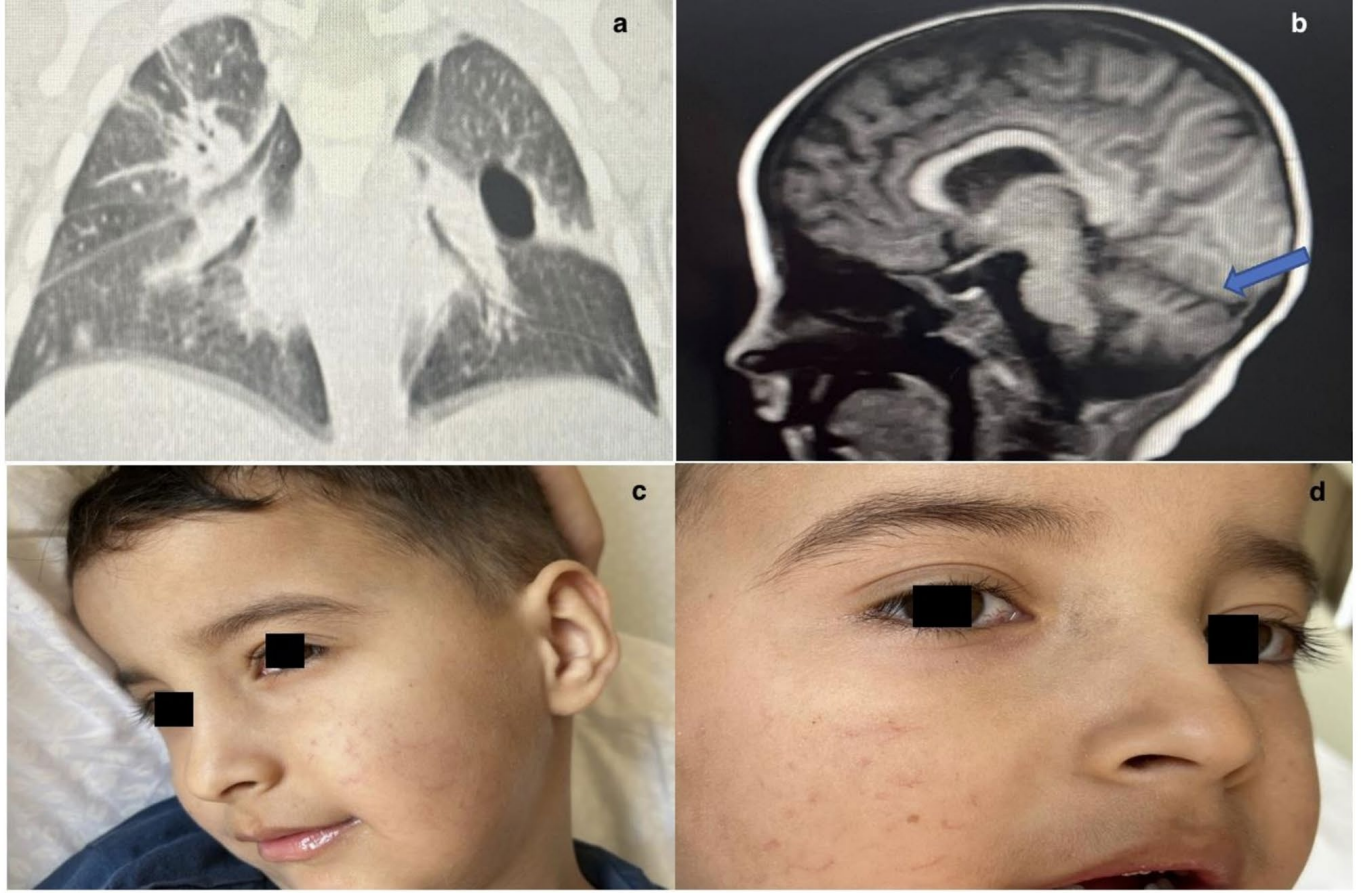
Imaging and examination findings of Patient 1. (a) HRCT image reveals a cystic appearance in the left lung, bilateral consolidation, and widespread atelectasis. (b) T1-weighted cranial MRI demonstrating cerebellar atrophy. (c, d) Telangiectasias visible on the cheeks and bulbar conjunctiva
